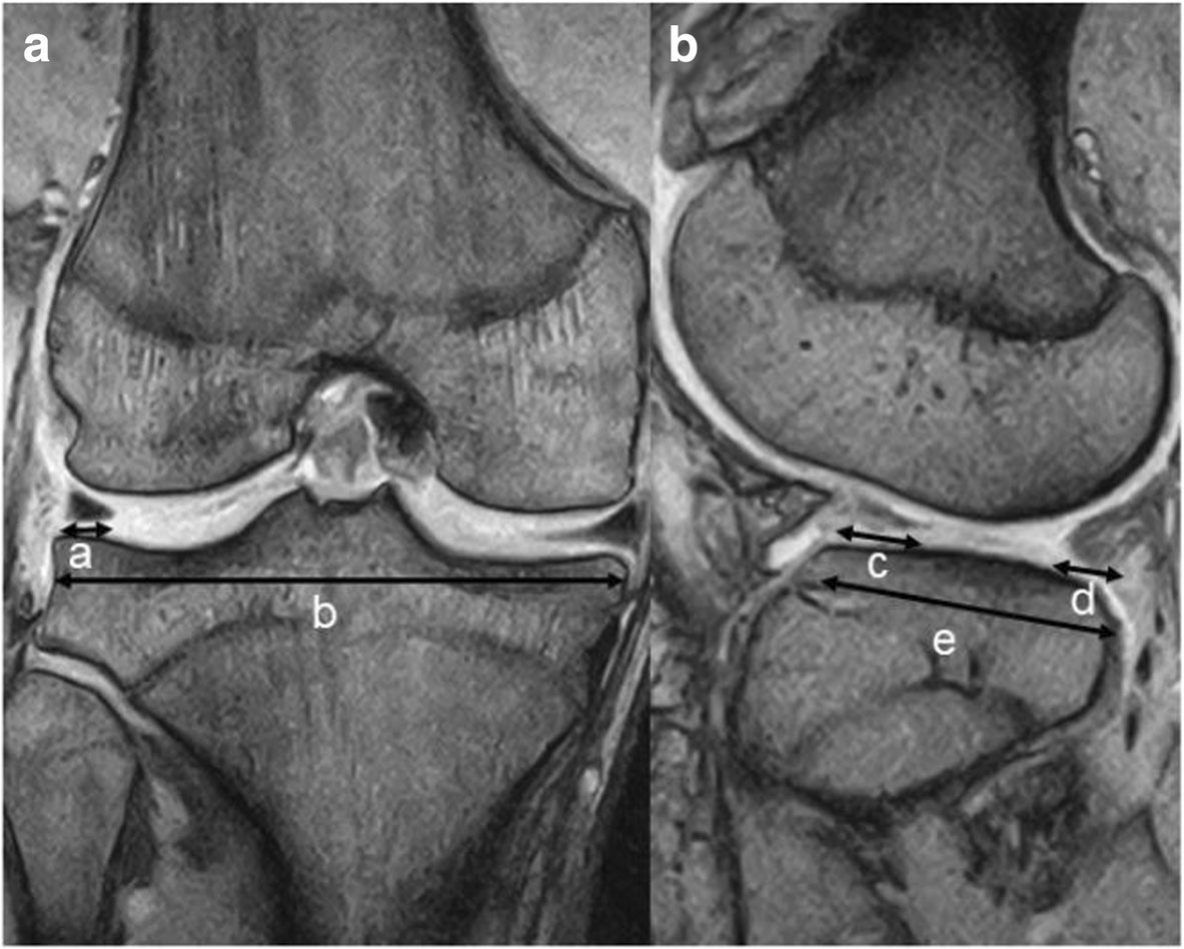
Diagnostic parameters of the DLM on an magnetic resonance imaging scan. Coronal plane (a). Lateral meniscal width; a mm. Ratio of the meniscus to the tibia; a/b × 100%. Sagittal plane (b). Percent coverage of the meniscus; c + d/e × 100%. DLM: discoid lateral meniscus
